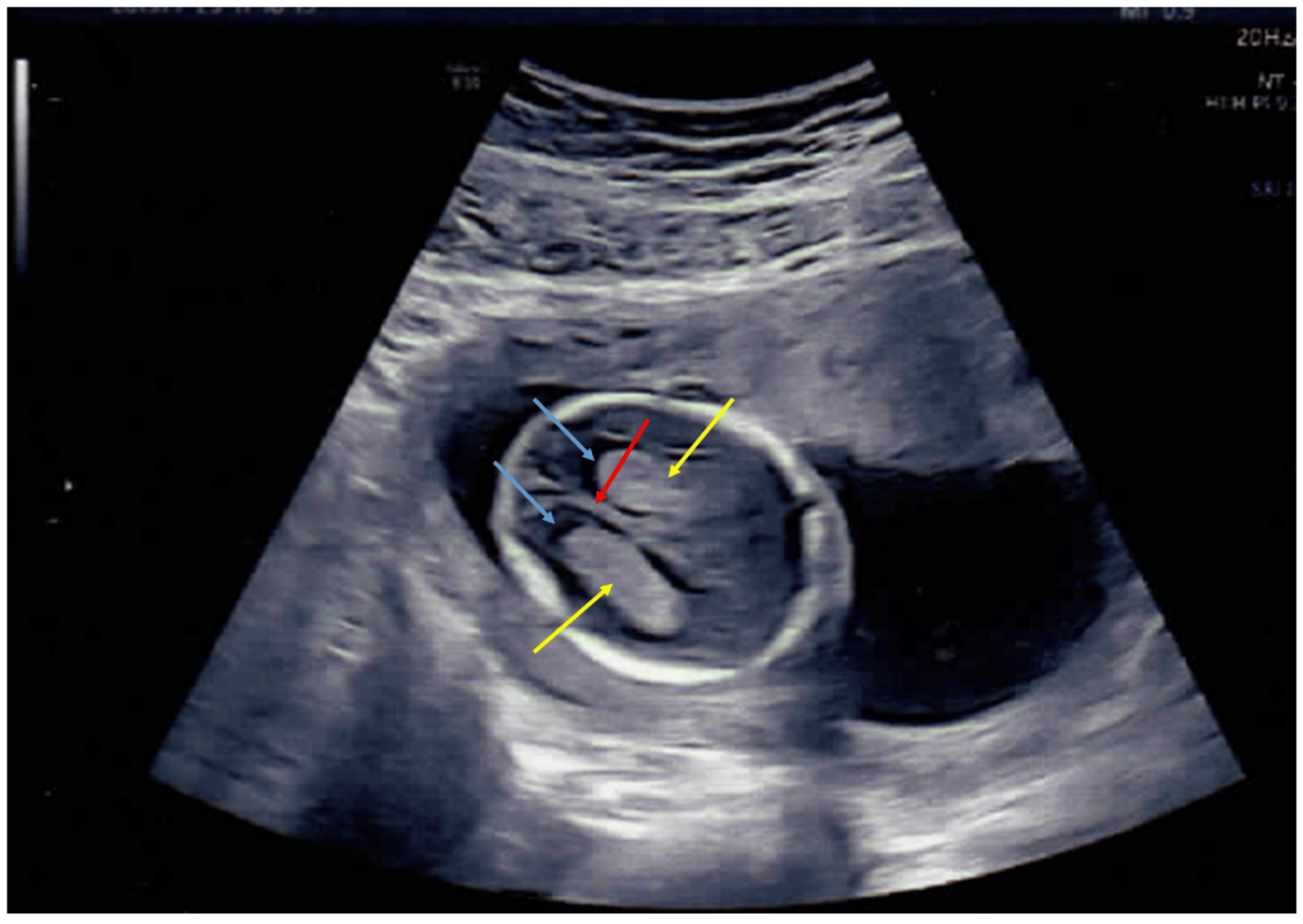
Antenatal ultrasound of the fetal skull around 15 weeks 3 days gestation in the couple's second pregnancy Antenatal ultrasound showing a transverse view of the the fetal skull at the level of the lateral ventricles showing normal intracranial anatomy around 15 weeks 3 days of gestation The choroid plexus (yellow arrows), lateral ventricles (blue arrows) and the midline falx cerebri are seen (red arrow).
